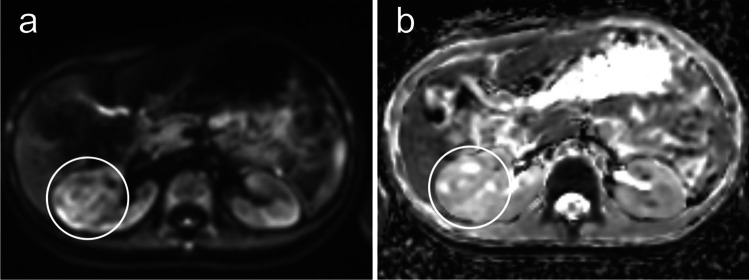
Axial magnetic resonance imaging of a 5-year-old boy. Absence of diffusion restriction of the metanephric stromal tumor (*circles* in a and b) in diffusion-weighted imaging, no apparent diffusion coefficient value reduction compared to the healthy kidney
